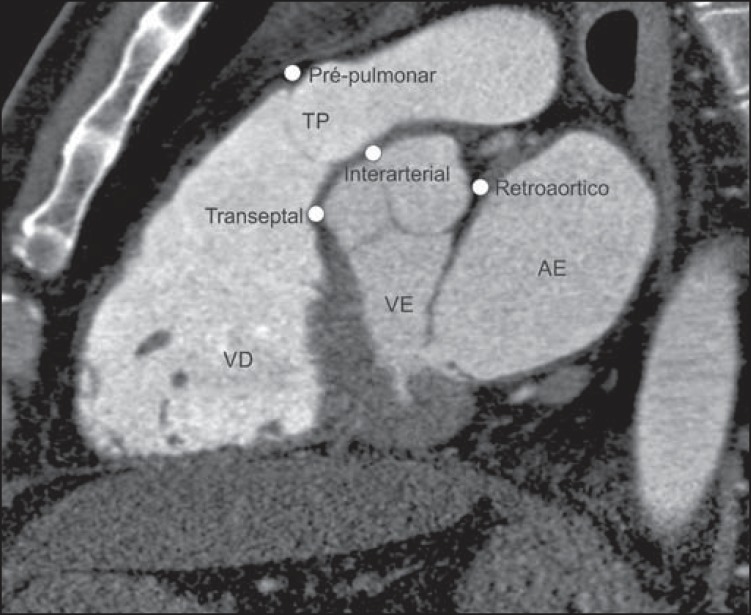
Triple rule-out chest computed tomography angiography with oblique sagittal
